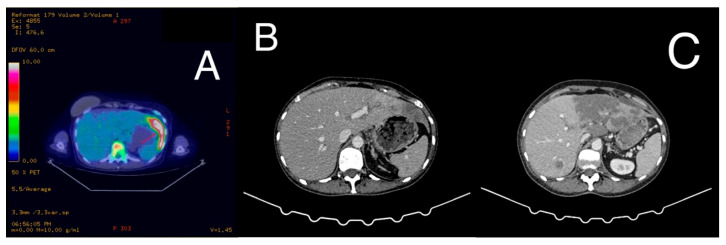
Radiologic assessment of hepatic metastasis before and during treatment. (A) Baseline 18F-FDG PET-CT showing intense metabolic activity within the left hepatic lobe metastasis. (B) Contrast-enhanced CT performed three months after initiation of chemoimmunotherapy demonstrating a reduction in hepatic metastasis size. (C) Subsequent CT revealing enlargement and new confluent hepatic lesions.
